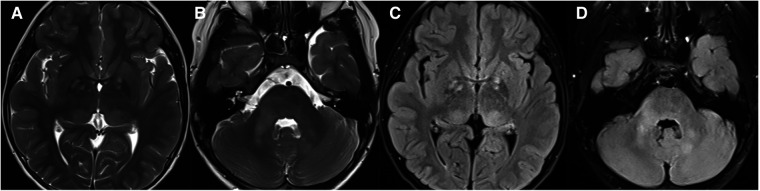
11-year-old boy with neurofibromatosis type 1: axial T2WI (A and B) and FLAIR (C,D) show hyperintensity in the bilateral basal ganglia, thalamus, brainstem, and cerebellum on T2WI/FLAIR.
